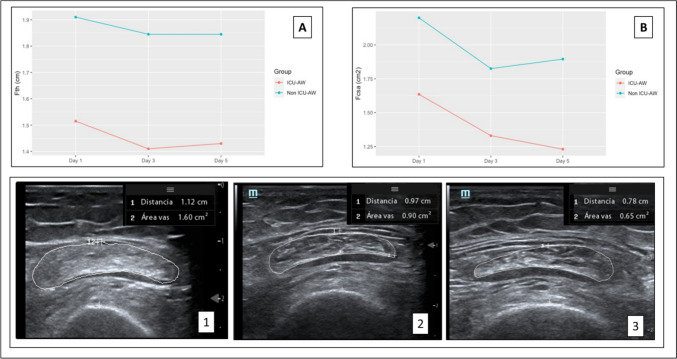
Evolution of ultrasonographic longitudinal changes in rectus femoris cross-sectional area (Fcsa) and the combined thickness of rectus femoris and vastus intermedius (F + VIth) muscles, measured via ultrasound on days 1, 3, and 5 post-ICU admission. A Evolutionary trend of Fth in ICU-AW vs. non-ICU-AW groups. B Evolutionary trend of Fcsa in ICU-AW and non-ICU-AW groups. In the bottom: Evolutionary trend of the two measurements (Fcsa and Fth) in ultrasound images of a patient on admission (Measure 1), day 3 (Measure 2), and day 5 (Measure 3) of ICU stay. Measurements were obtained using a standardized protocol using a linear transducer with measurements taken at the lower third of the quadriceps femoris. The transducer was then positioned in a transverse axis with respect to the extremity at the level of the previously marked point, forming a 90-degree angle with respect to the extremity. Potential confounding factors such as pre-existing muscle conditions were excluded (see text for complete list of exclusion criteria)
